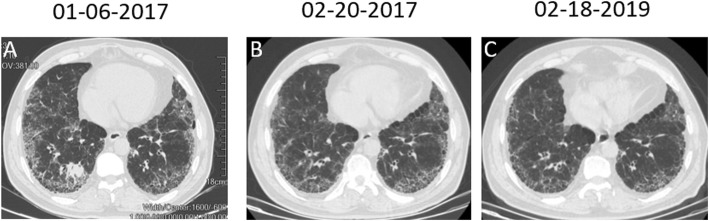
Serial chest computed tomography (CT) scans showing complete regression of the tumor. a The chest CT scan at first presentation revealed a tumor in the right lower lobe. b The follow-up chest radiograph revealed complete regression of the tumor 1 month after the first cycle of chemotherapy. c Complete disappearance of the tumor 2 years after the first presentation
